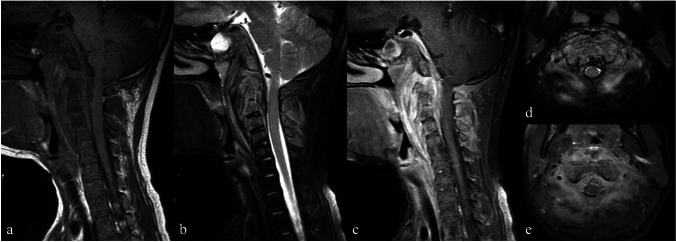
Magnetic resonance images of an 11-year-old female patient with actinomycosis spondylitis involving the cervical spine. Sagittal T1-weighted (a), short tau inversion recovery (b), fat-suppressed contrast-enhanced T1-weighted (c), axial T2-weighted (d), and contrast-enhanced T1-weighted (e) images show diffuse enhancement of C1–C5 vertebrae, with thickened and enhanced soft tissue at perivertebral and anterior epidural regions. The cervical discs are preserved without gross destruction. Diffuse enhancement of the clivus, representing skull base osteomyelitis, along with retroclival, epidural enhanced soft tissue and reactive fluid; partially opacified sphenoid sinus is also noted
